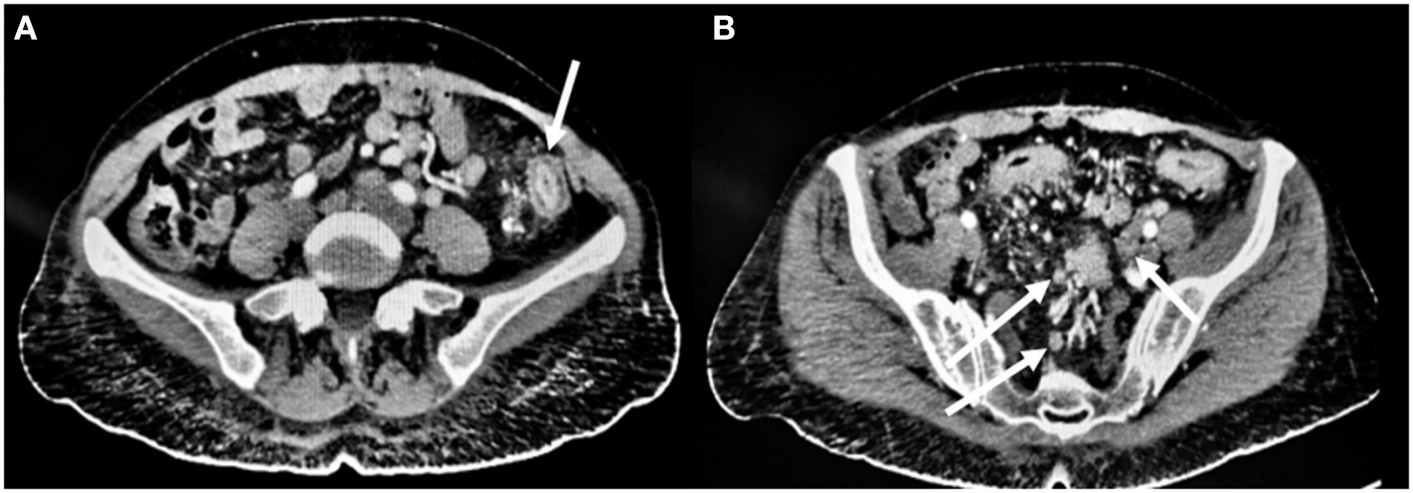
Pre-operative CT scan findings. (A) Circumferential, symmetrical wall thickening with fold enlargement at the level of the sigmoid colon (arrow) along with inhomogeneous attenuation and proliferation of perirectal fat. (B) Concomitant multiple reactive lymph nodes (arrow) in the mesentery, perirectal and parasigmoid tissue.
